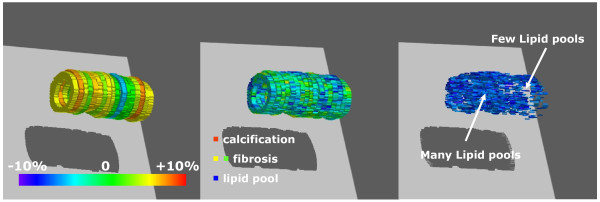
Three-dimensional intravascular ultrasound images. (Left) Color-coded map of plaque volume response. Each color corresponded to plaque volume compression and expansion (percentage per 10 mmHg). Light green to orange signified plaque expansion, and dark green to blue indicated plaque compression during cardiac cycle. (Middle) Three dimensional integrated backscatter intravascular ultrasound (3D IB-IVUS) color-coded map that showed plaque components. (Right) 3D IB-IVUS color-coded map that depicted only lipid pool at the same lesion as the middle image. Note that plaque area response in the lesion with relatively many lipid pools (left side of the segment) was greater than that in the lesion with relatively few lipid pools (right side of the segment).
